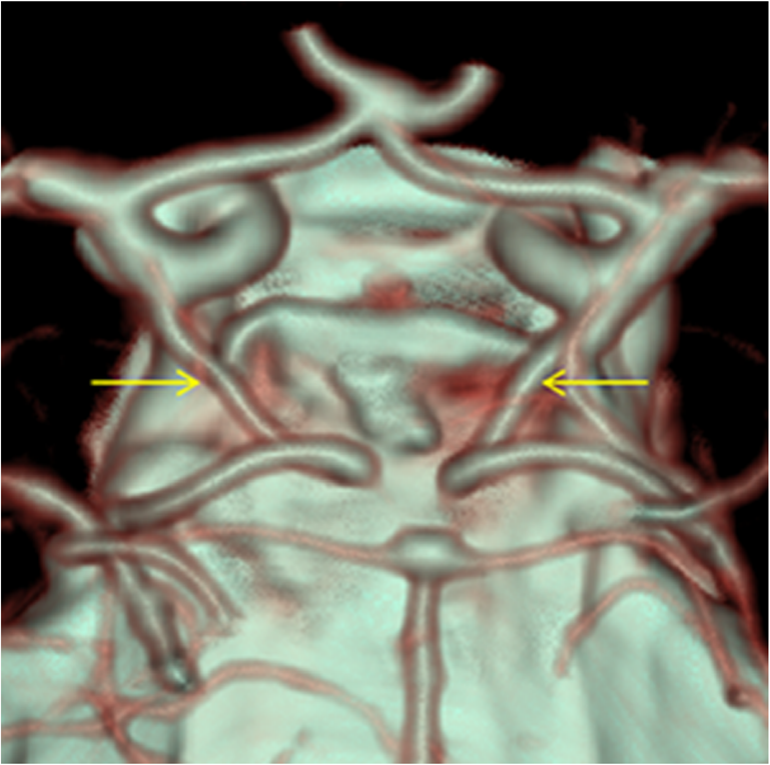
CTA (VRT 3D reconstructions) shows a bilateral foetal Pcom (*yellow arrows*)
